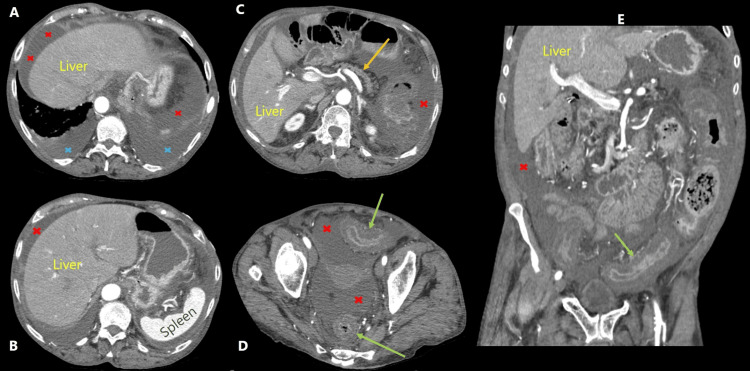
Contrast-enhanced computed tomography images (A-D) Axial images demonstrating ascites (red x), pleural effusion (blue x), a normal-appearing pancreas (yellow arrow), and thickened sigmoid and rectum (green arrows); (E) coronal image depicting ascites (red x) and thickened sigmoid (green arrow).
